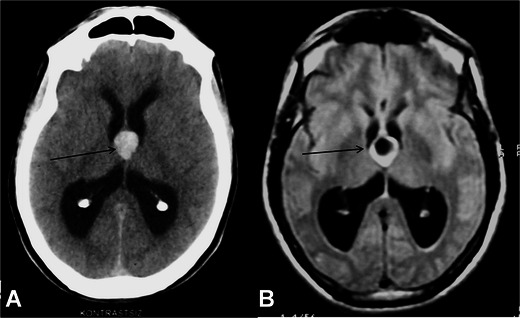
A 46-year-old male patient with a colloid cyst. Axial noncontrast-material enhanced CT image demonstrates hyperdense lesions located in the foramen of Monro sized 2 cm in diameter (*arrow*, A). The lesion is hypointense in FLAIR images, which indicates the proteinous content of the cyst (*arrow*, B)
